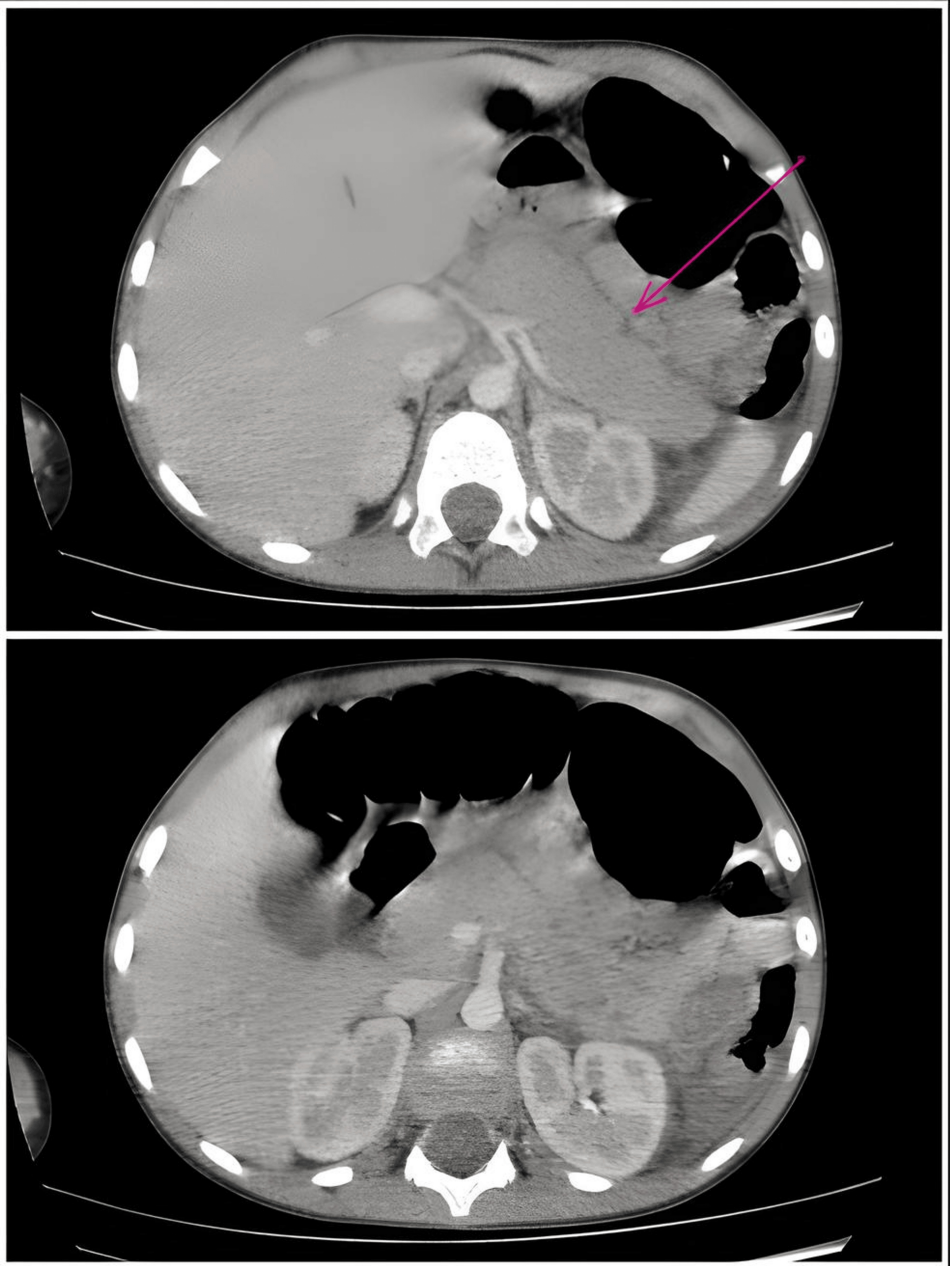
Axial slices of an abdominal CT scan performed in a five-year-old boy with acute pancreatitis, Balthazar grade E.
